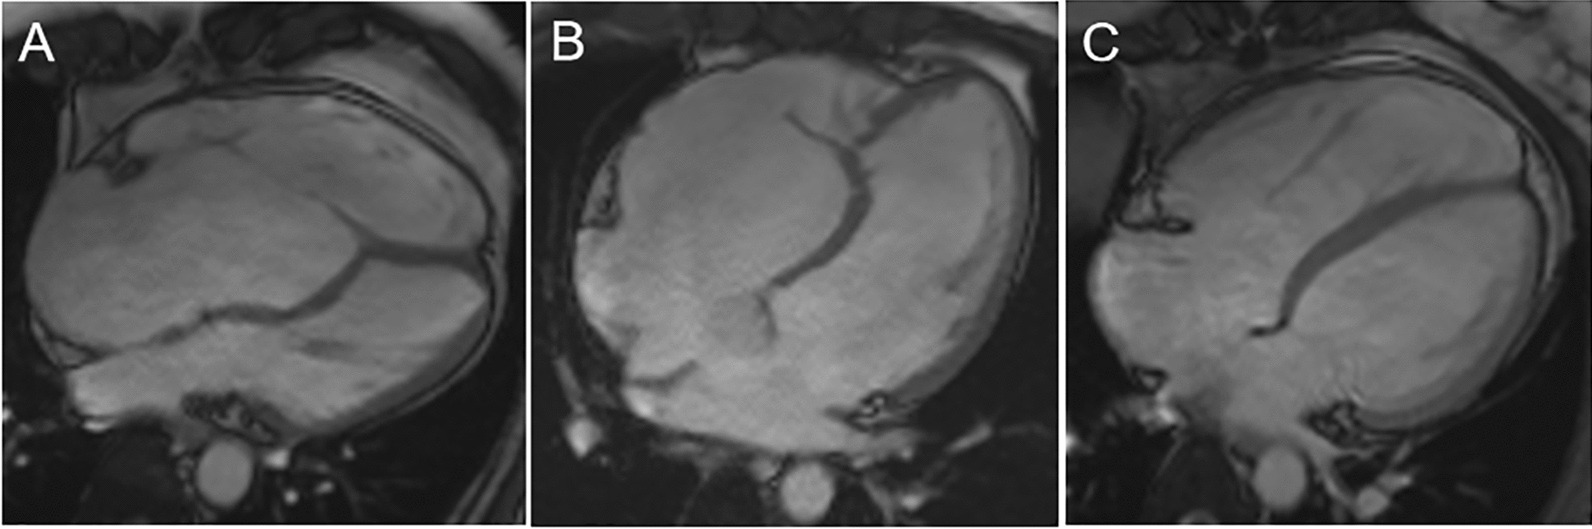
Representative four-chamber cardiac magnetic resonance images at the end-diastolic phases in EA patients without ASD (A), EA patients with ASD (B), and ASD patients (C)
